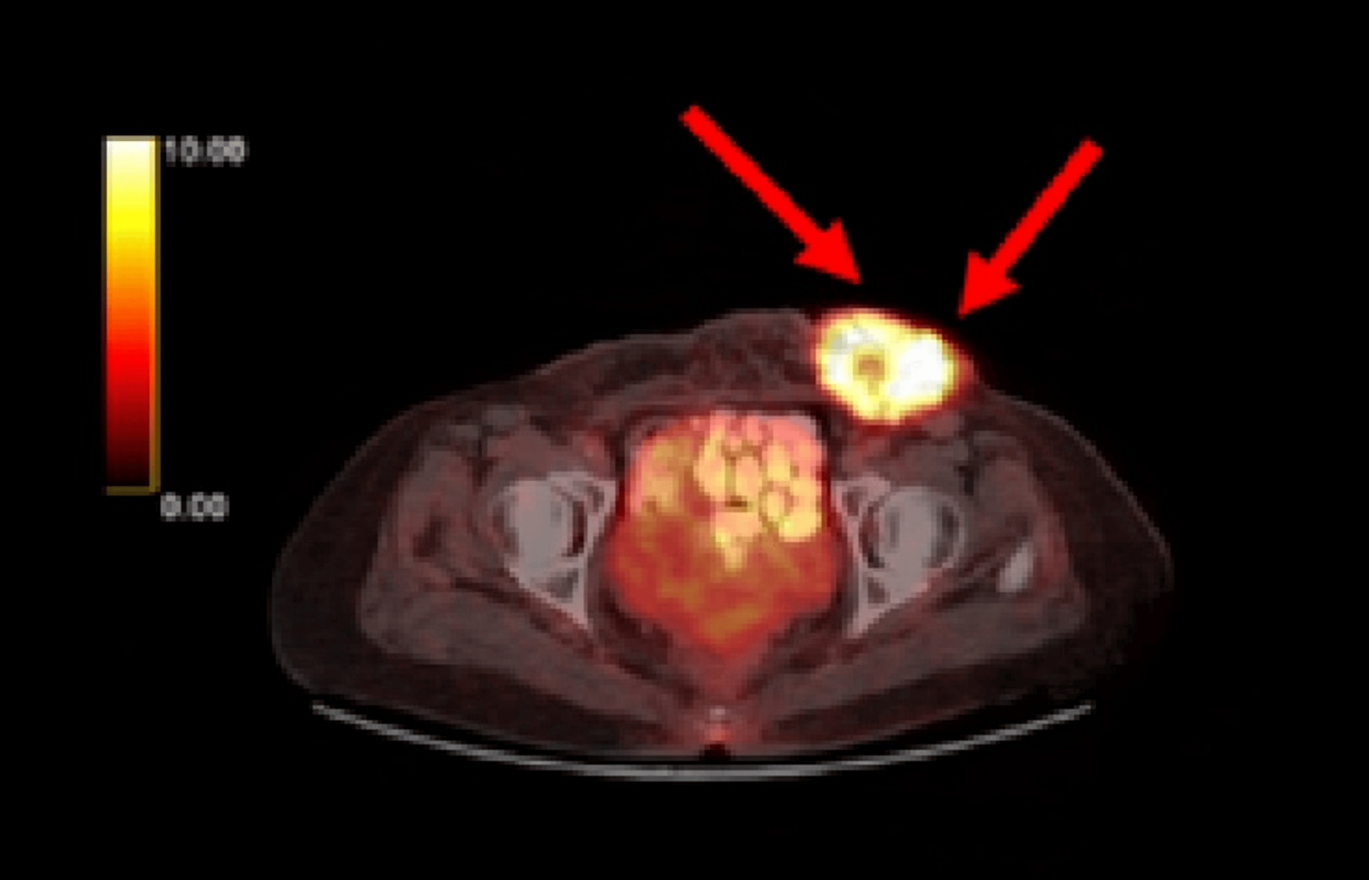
Axial PET/CT image showing a hypermetabolic subcutaneous lesion in the left inguinal region (red arrows) with an SUVmax of 13.7, consistent with metabolically active metastatic disease PET/CT: positron emission tomography/computed tomography
